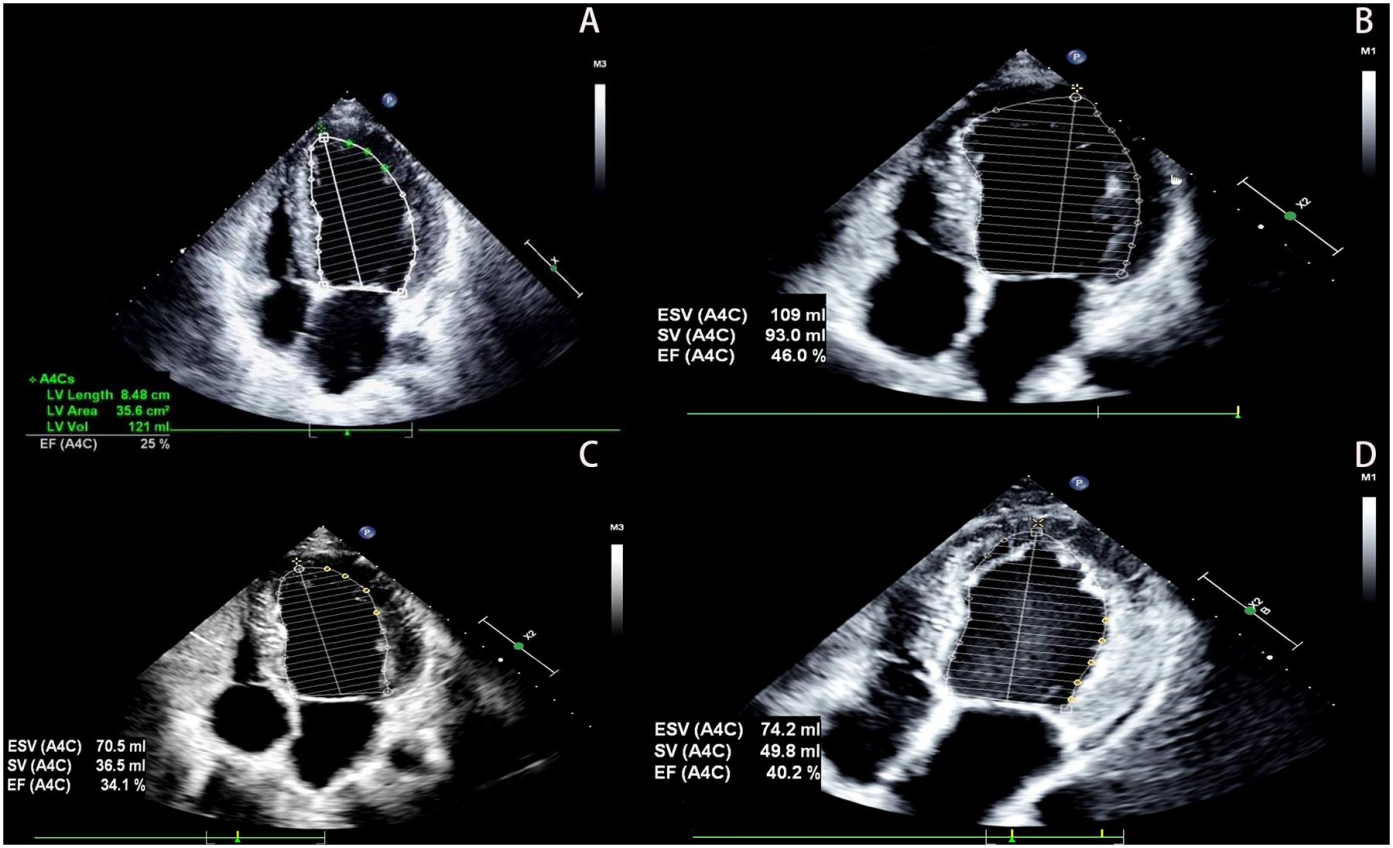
The patient's echocardiography results. (A) Show the echocardiographic result of the patient's heart at hospitalization (2022). (B) Show the echocardiographic result of the patient's heart at 1 year follow-up (2023). (C) Show the echocardiographic result of the patient's heart at 2 years after discharge, post-infection (2024). (D) Show the echocardiographic result of the patient's heart at 3 years follow-up (2025).
